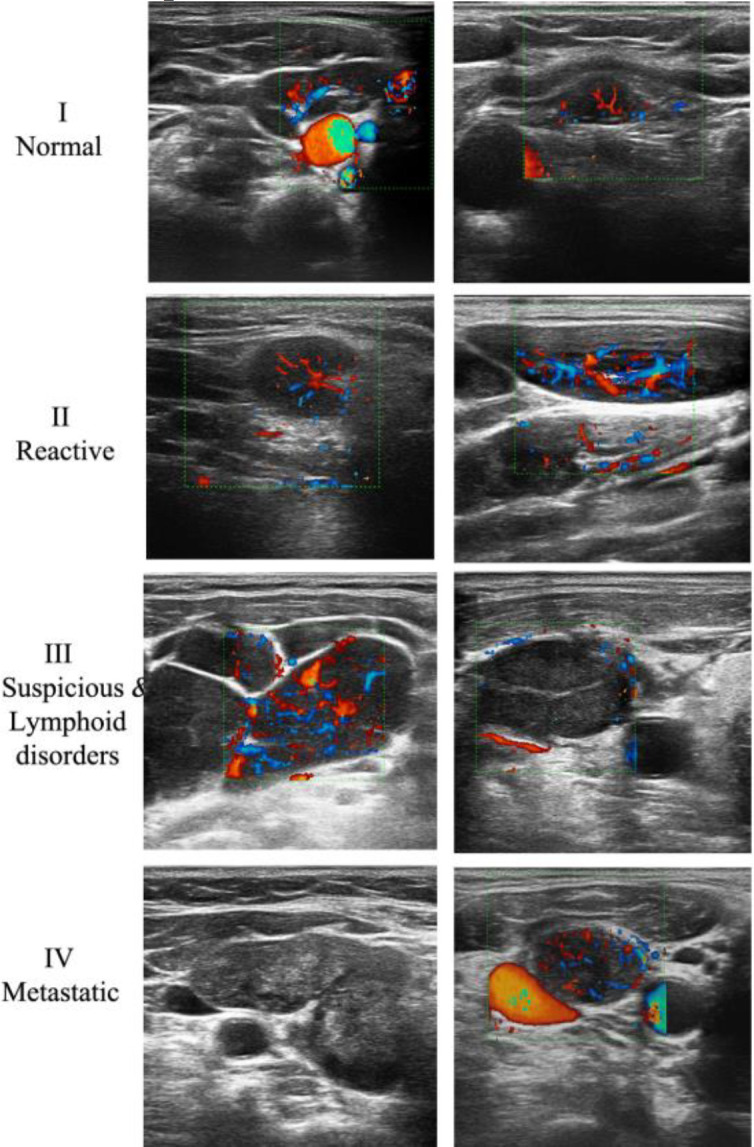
Adenopathy Reporting and Data Systems classification in ultrasound images; the ultrasound appearance of normal lymph nodes (I) and adenopathies (II-IV) according to the proposed categories (A-RADS): In group I, it shows normal lymph nodes in the jugulo-digastric area (right) and other cervical levels (left). In group II, it shows short axis (right) and long axis (left) of reactive adenopathies with increased hilar hyper-vascularity. In group III, it shows huge hypoechoic adenopathies of non-Hodgkin lymphoma (right) and necrotizing granulomatous lymphadenitis with decreased vascularity (left). In group IV, it shows adenopathy with iso-echoic cortex (right) or peripheral non-hilar vessels (left) in two different cases with squamous cell carcinoma
